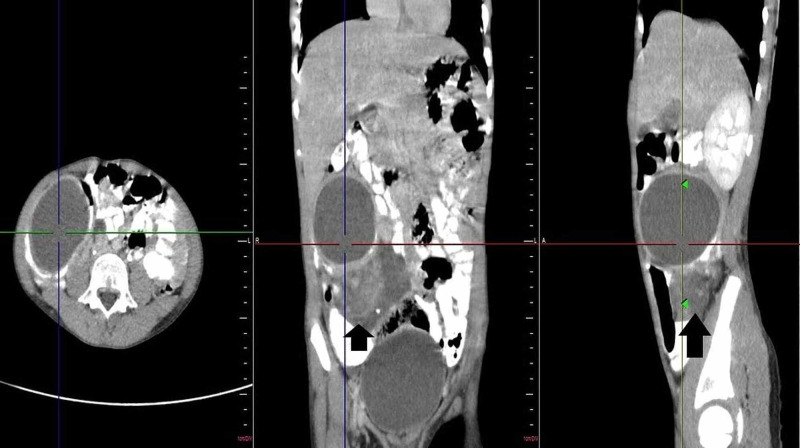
Contrast-enhanced CT image with the arrowhead directed towards the heterogeneously enhancing solid lesion.
